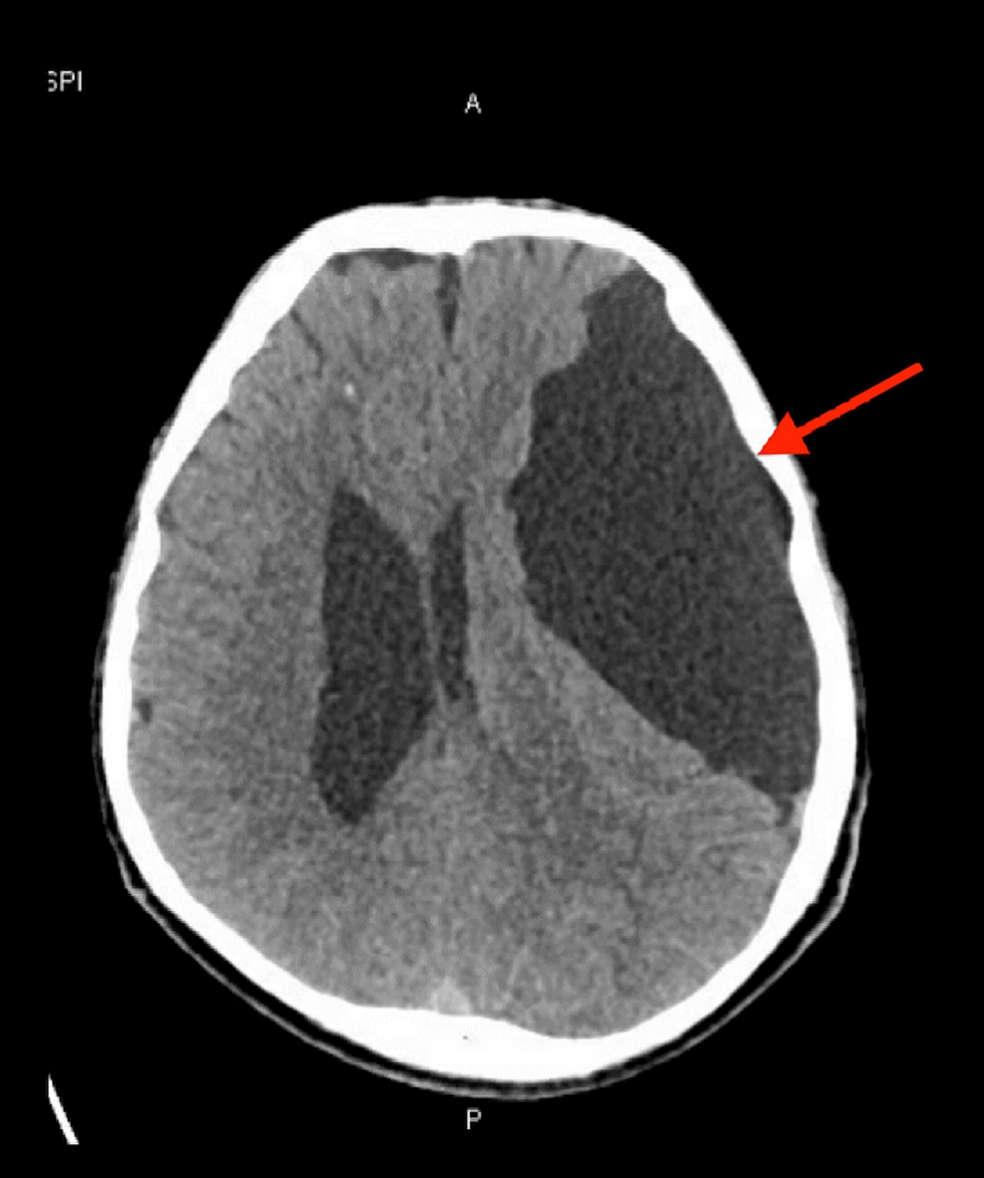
Initial CT head scan showing a large CSF density extra-axial cystic lesion along the left cerebral convexity consistent with an arachnoid cyst, causing a mass effect and midline shift to the right CT: computed tomography; CSF: cerebrospinal fluid
